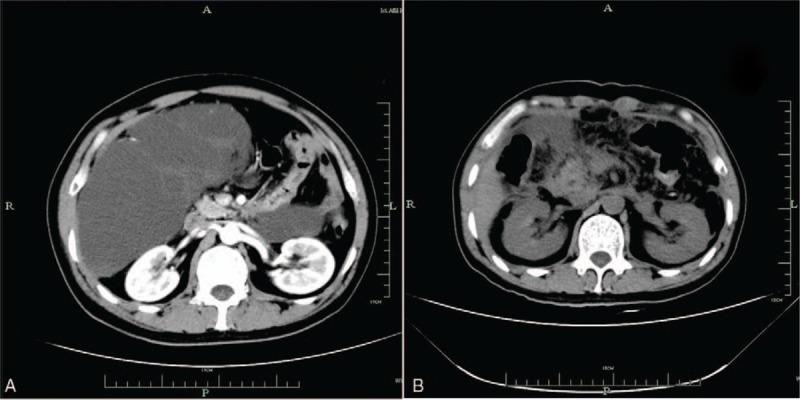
The CT scan imaging of the lymphangioma before and after the operation. (A) Before the operation, the CT scan demonstrated large cystic lesion with thin walled cavities in the abdomen. (B) The CT image after distal pancreatectomy and splenectomy.
